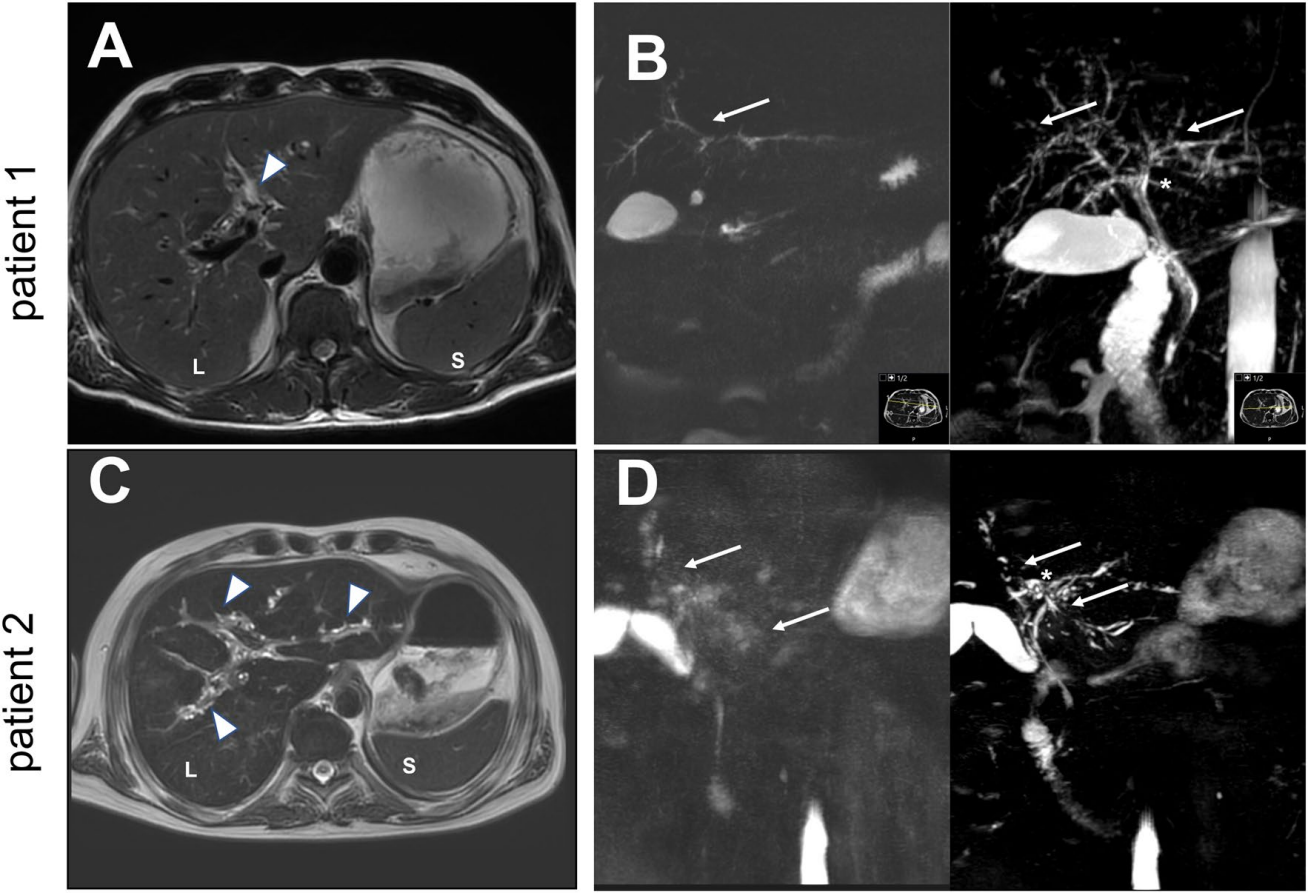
MRI A and C and MRCP B and D scan of patient 1 (top) and patient 2 (bottom), showing structural abnormalities of the bile ducts, such as intrahepatic multifocal strictures (arrows), bile duct ectasia (*), ductal wall thickening (arrow heads), irregular beading, and cast formation in the proximal common bile duct. *L* liver, *S* spleen
